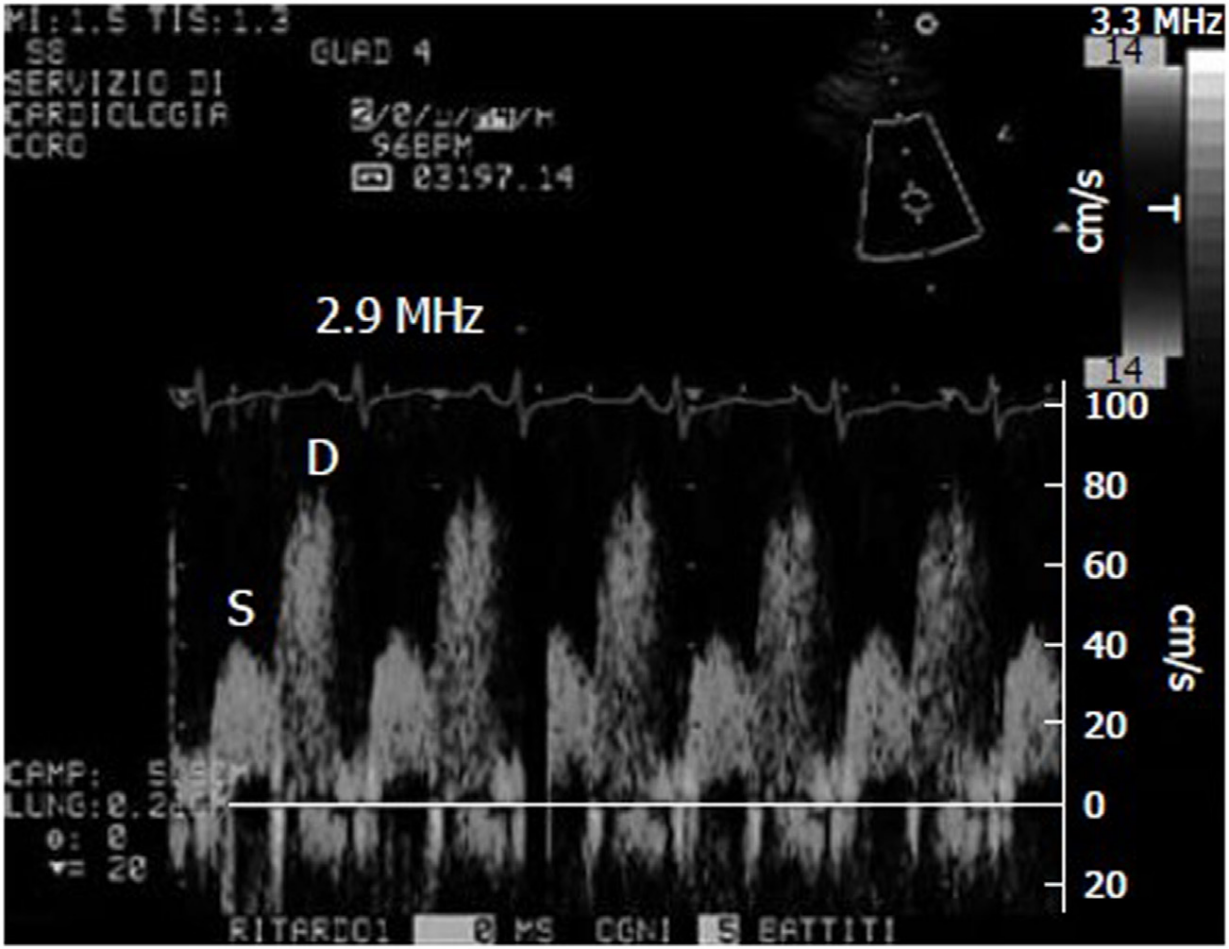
Example of coronary flow Doppler signal during dypiridamole-induced hyperemia. S, systolic flow; D, diastolic flow.
